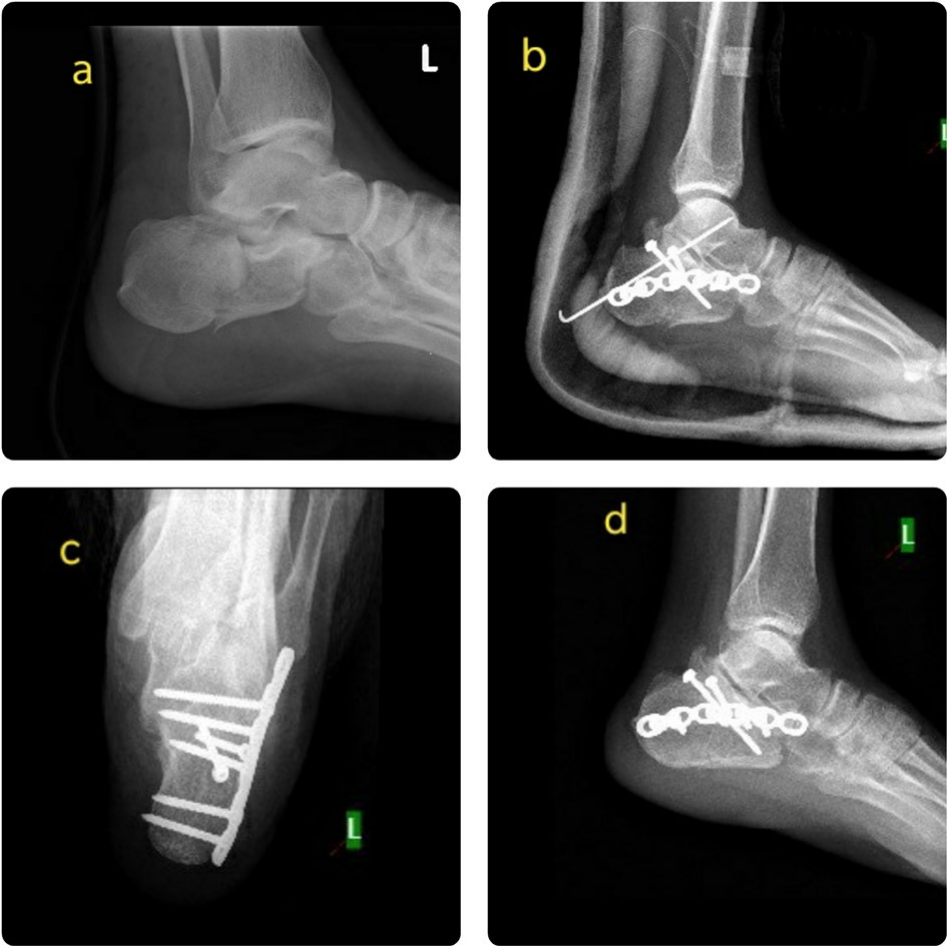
Calcaneal fracture treated by operative method. (a) Preoperative radiograph, (b) post-operative radiograph, (c,d) radiographs at one year follow up.
